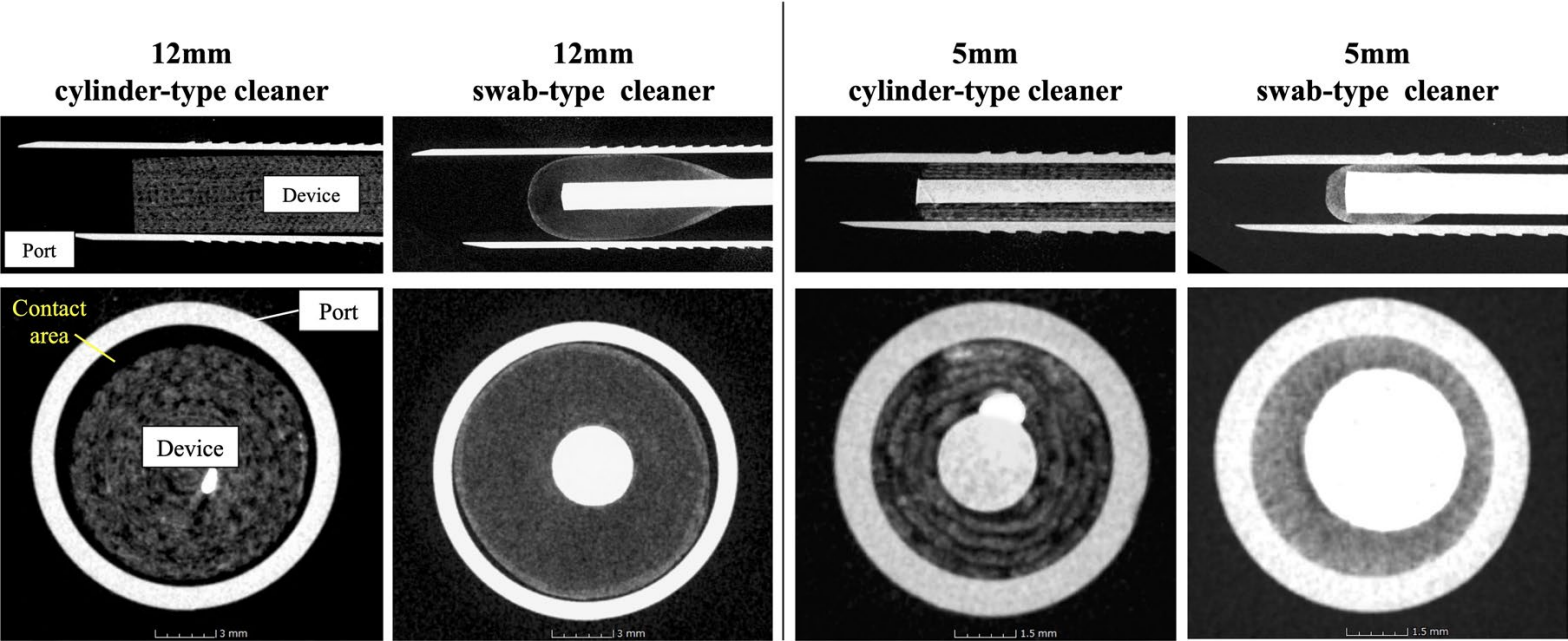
Visualization for contact area of dedicated cleaning devices. Sagittal section and axial section of microfocus CT scans with each device inserted into the port are shown. The contact area (yellow text) is the space between the device and the port sleeve (Color figure online)
